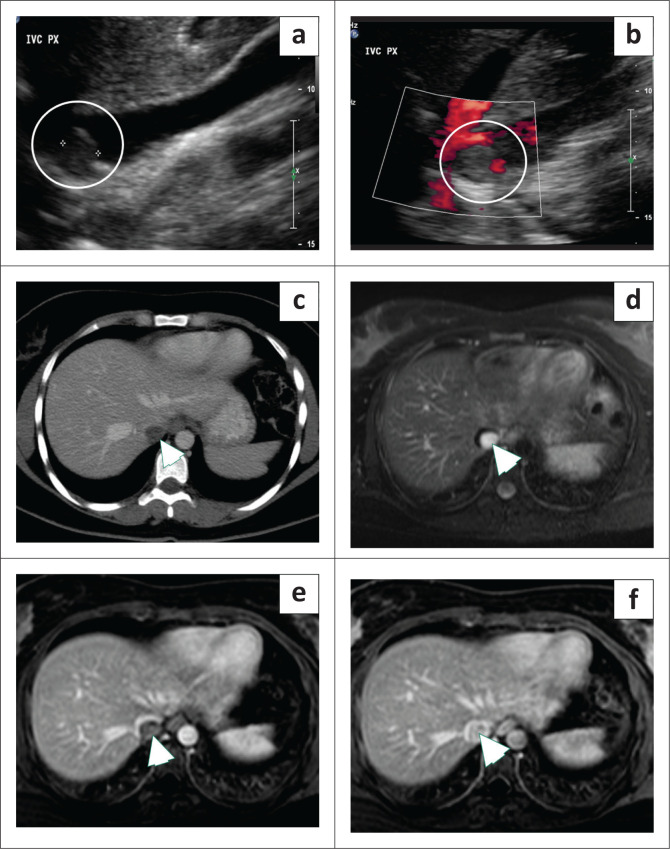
A 36-year-old female with acute abdominal pain related to cholelithiasis and an incidental inferior vena cava (IVC) finding on upper abdominal ultrasound. (a) Greyscale ultrasound image showed a 1.5 cm hypoechoic lesion (circle) within the IVC lumen. (b) The colour Doppler image shows no significant internal flow within the lesion (circle). (c) Contrast-enhanced CT of the abdomen in the portal venous phase did not reveal significant enhancement (arrowhead). (d) Axial T2-weighted images of the abdomen show hyperintense signal within the IVC (arrowhead). Dynamic contrast-enhanced MRI (e) portal venous phase and (f) delayed sequence acquired at 5 min after the injection demonstrate progressive enhancement with centripetal fill in suggesting the diagnosis of an IVC haemangioma (arrowhead).
